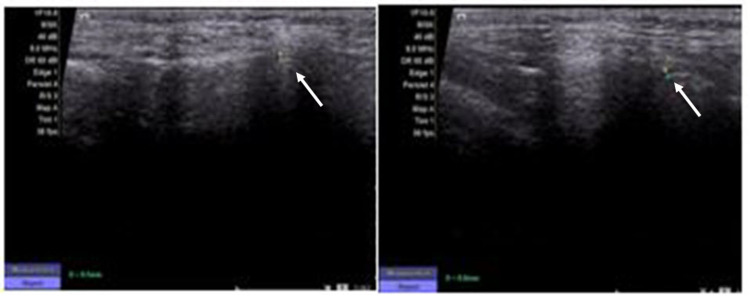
Ultrasound images taken at T0 (left) and T1 (right) to demonstrate the dimensional changes of the condylar cartilage after twin-block therapy
